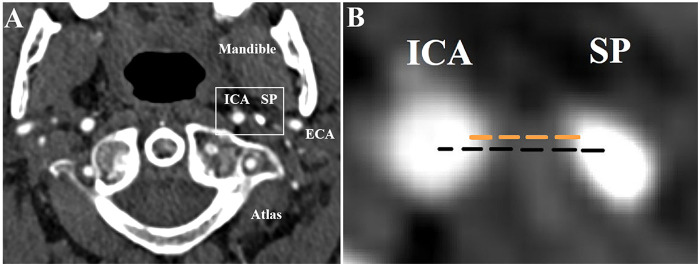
A. Axial CTA images and a detailed view of a healthy control subject, displaying the general anatomy on the axial view. B. Enlarged section marked with a white rectangle on image A, showing different approaches used in SP-ICA measurement (the shortest marginal SP-ICA distance is marked with orange dotted lines, the shortest intercentric SP-ICA distance with black dotted lines) CTA, computed tomography angiography; ICA, internal carotid artery; SP, styloid process; ECA, external carotid artery.
